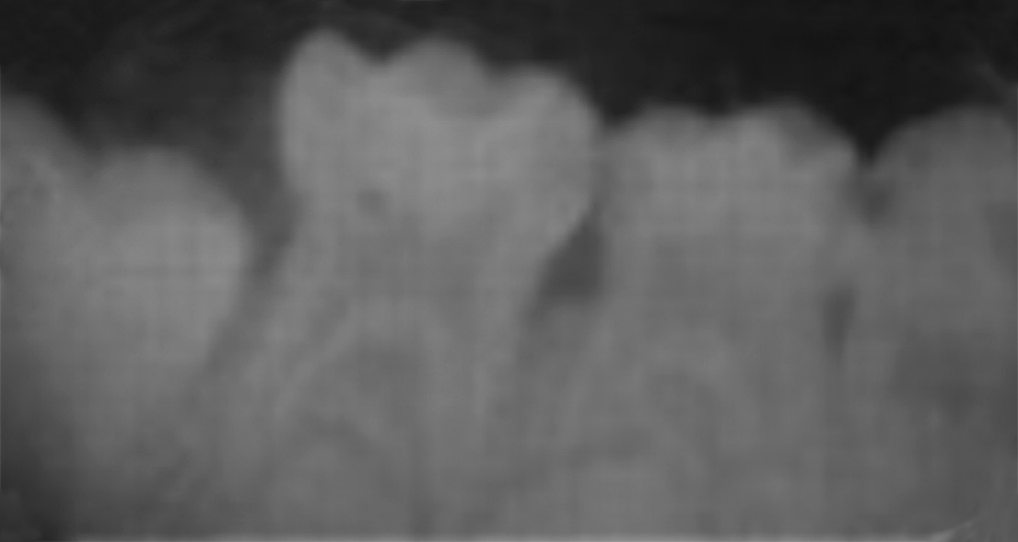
Case selection for pulpotomy: Radiographic criteria
